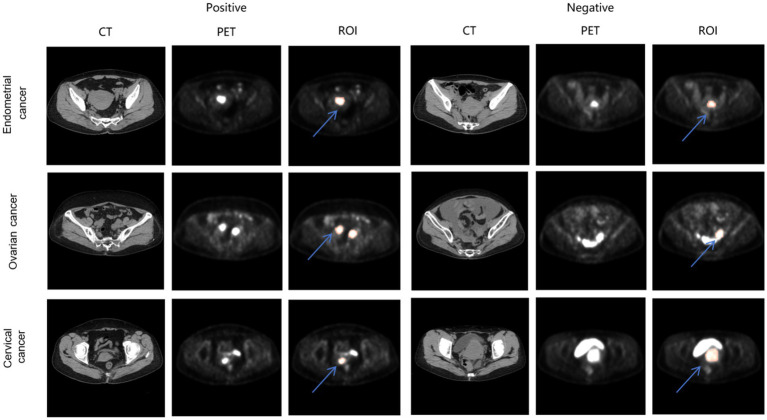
PET/CT images of endometrial cancer, cervical cancer, ovarian cancer in different TP53 states. The red region indicated by the blue arrow represents the annotated ROIs.
